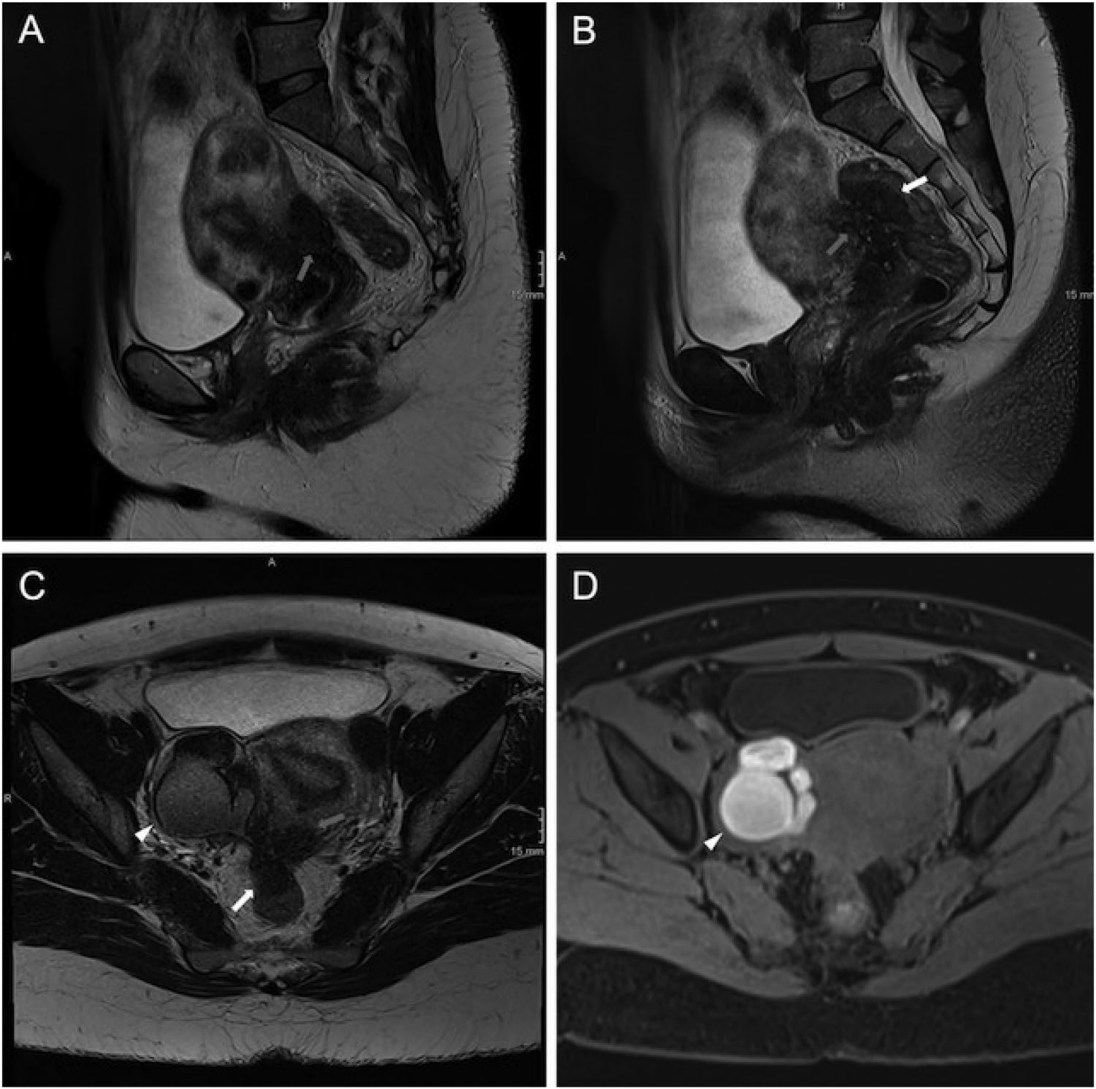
38-year-old patient with external adenomyosis of the posterior uterine wall (grey arrow in A–C) and deep endometriosis of the rectum and rectosigmoid junction (white arrow in A–C) with T2w-hyperintense foci, a T1w-hyperintense endometrioma of the right ovary (white arrowhead in C and D) with characteristic «T2-shading-sign» and adhesions between the right ovary, uterus and bowel A–C: high resolution 2D T2-weighted TSE images, D: native 3D T1-weighted GRE image with fat-suppression. mr#Enzian score: O0/2 T0/3 C3 FI FA(e)
